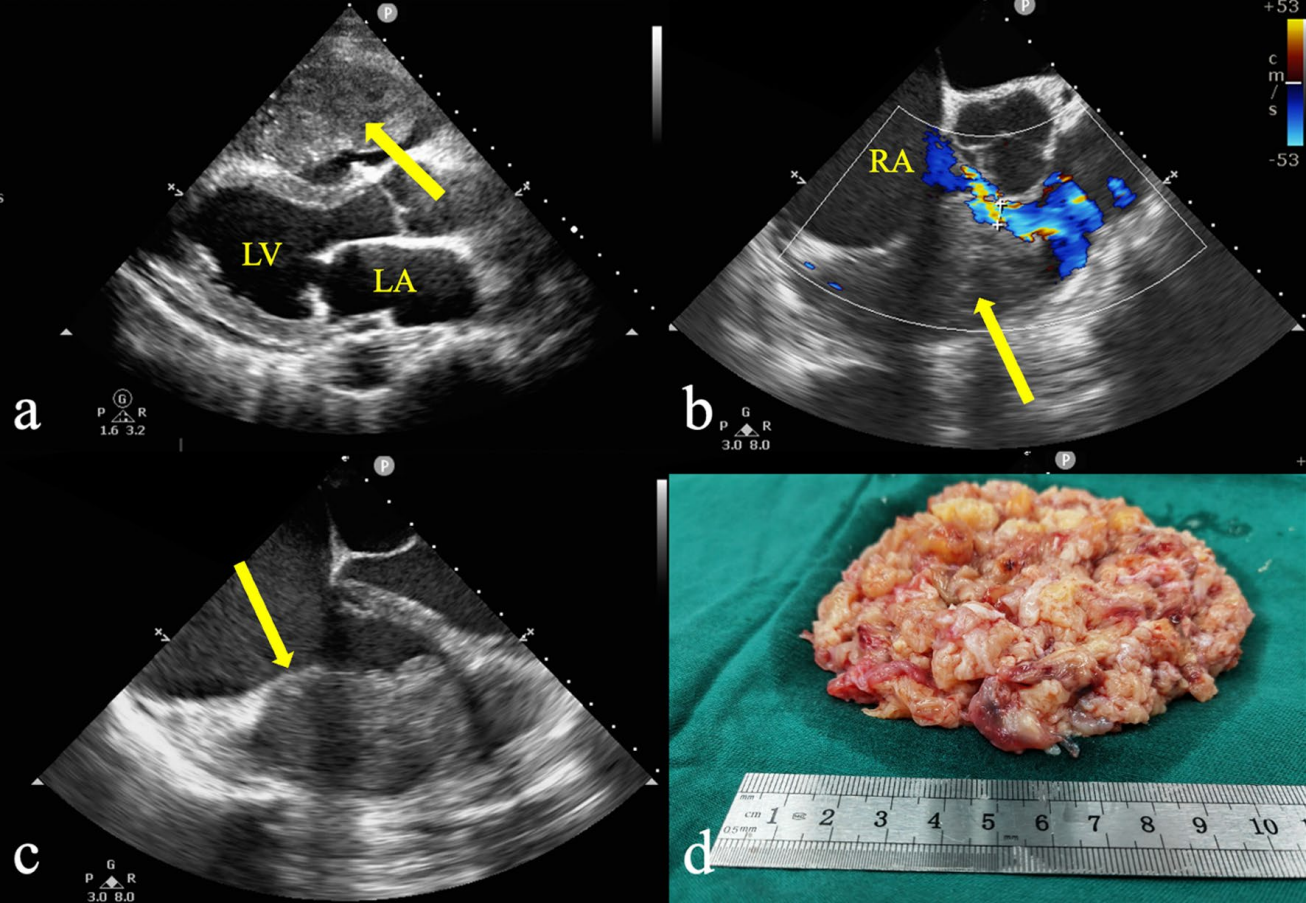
a Transthoracic echocardiography showed a huge mass (yellow arrow) occupying the inflow tract and most of the right ventricular cavity. b In the mid-esophageal RV inflow–outflow view, the mass was closely related to the right ventricular free wall (yellow arrow) and the patient had only a narrow gap of approximately 6 mm between the tumor and the supraventricular crest to provide forward blood flow during diastole. c The mass fused and wrapped with the anterior and posterior tricuspid valves, and the demarcation between the tumor and the myocardium was not clear (yellow arrow). d The excised fragmented tumor tissue. The findings of the pathological examination suggested leiomyosarcoma. *LA* left atrium, *LV* left ventricle, *RA* right atrium
